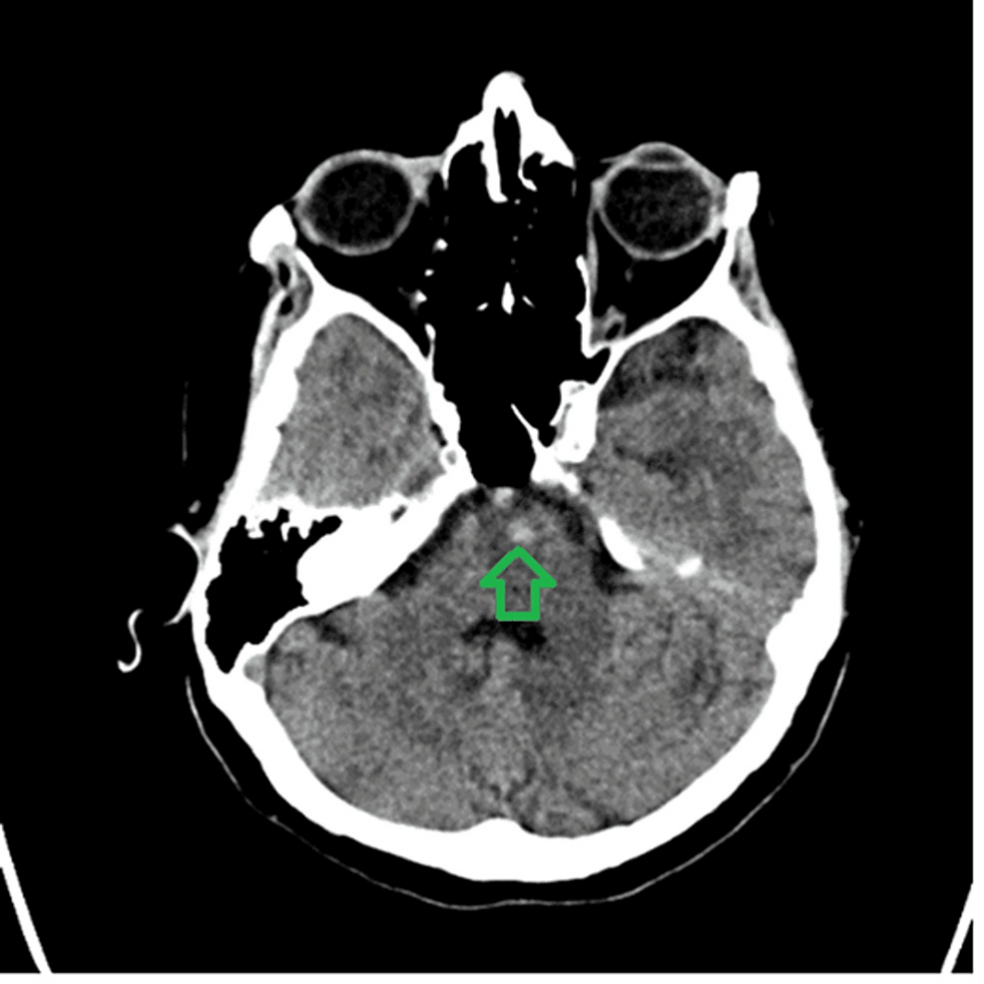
CT brain showing hyperdensity in anterior pons with 55 Hounsfield units suggestive of haemorrhage CT: computed tomography
